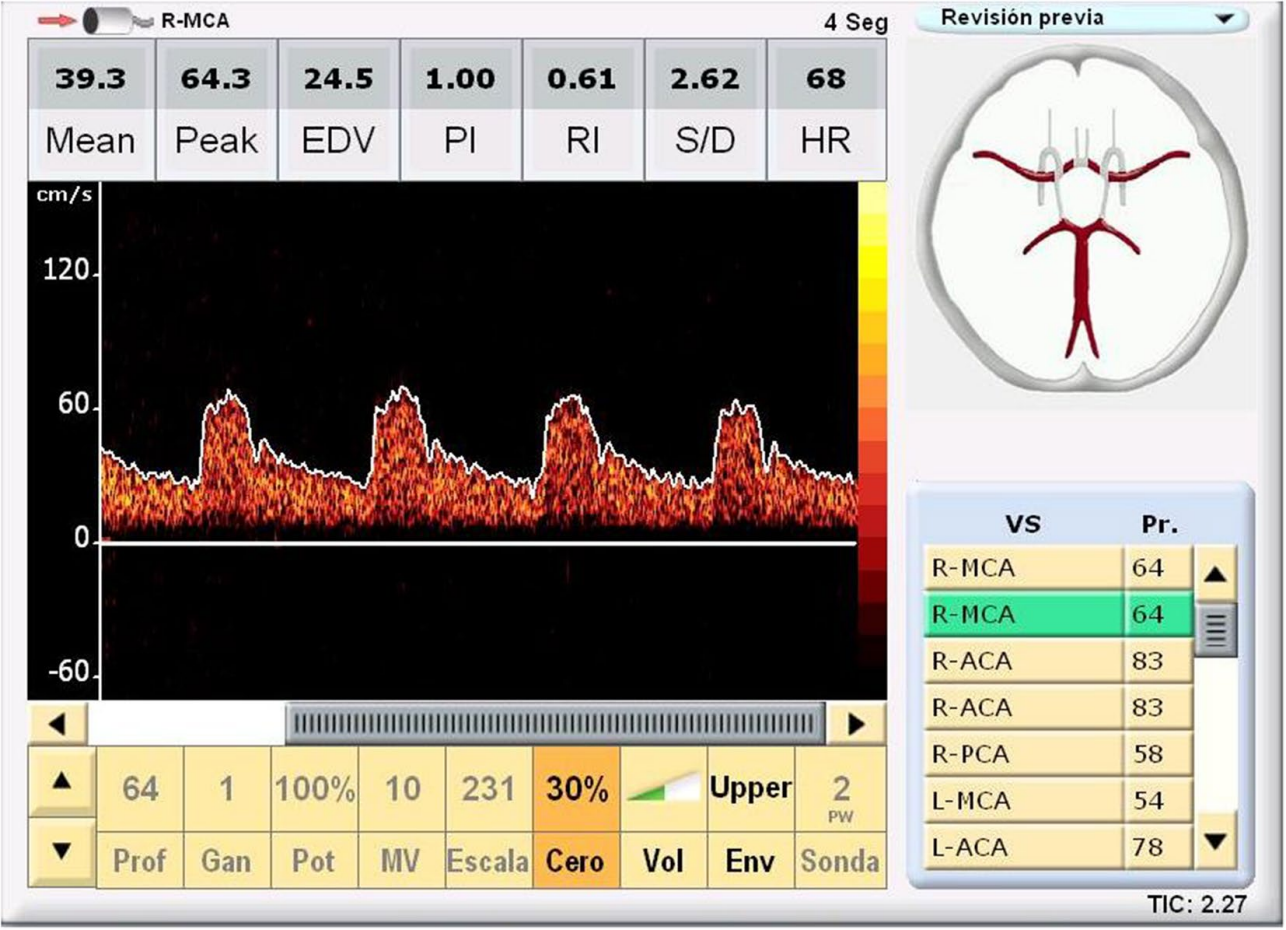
Screen of the Transcranial Doppler Study Performed in a Study Participant. Image showing the parameters evaluated for each artery (in this particular image, the right middle cerebral artery)
